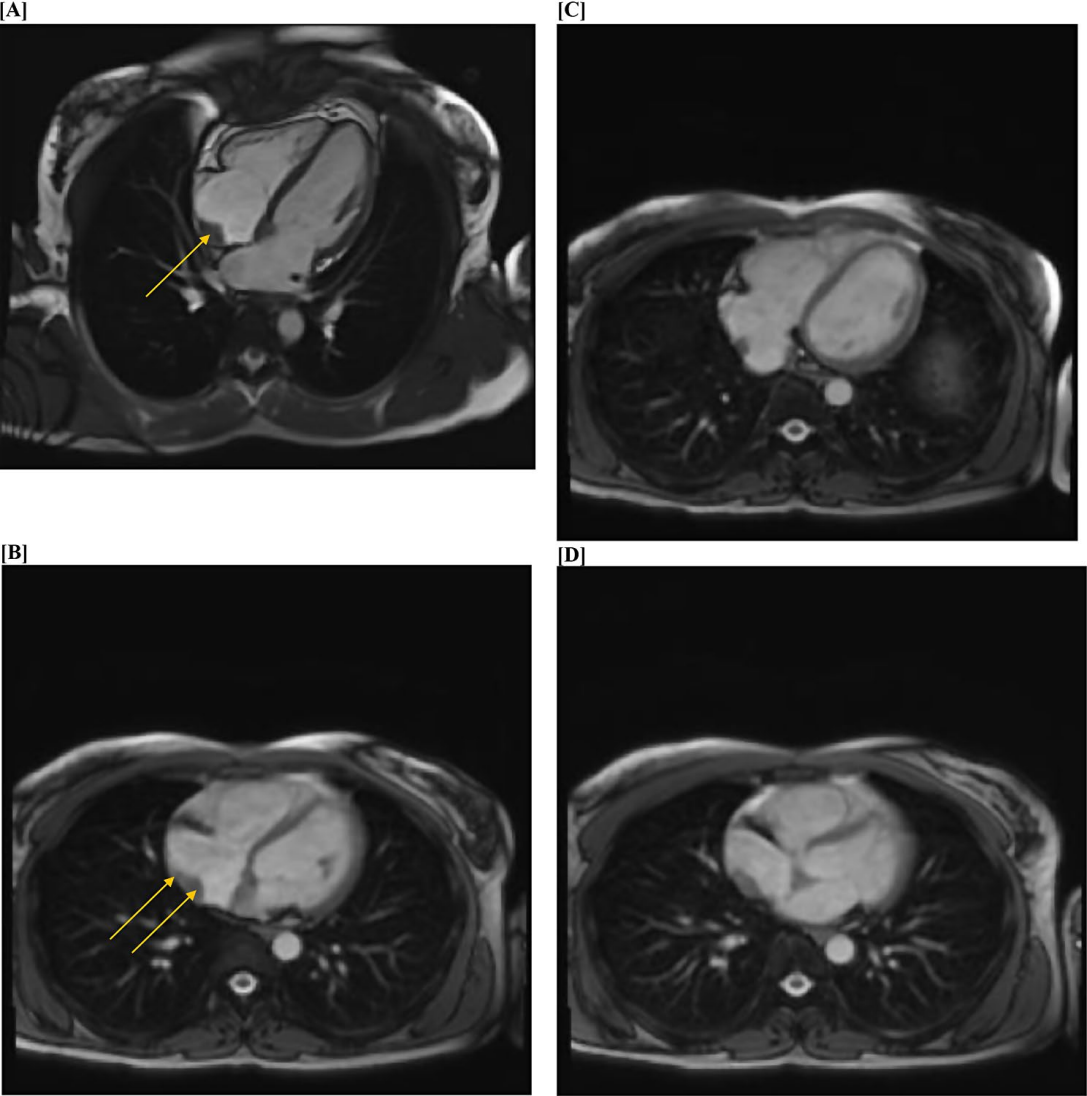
Cardiac MRI (A-D)
